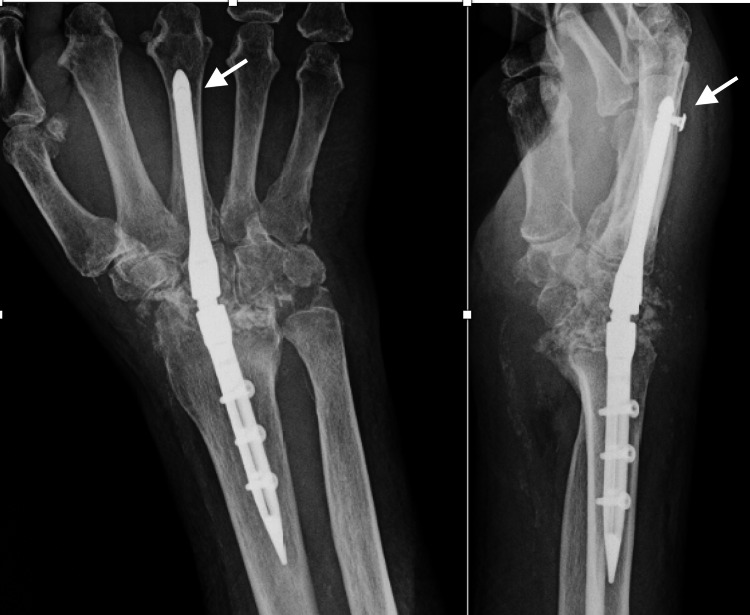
Immediate postoperative radiographs of Case 2 Posteroanterior and lateral views after total wrist arthrodesis using IMPLATE intramedullary nail and proximal row carpectomy. The white arrows show the proper positioning of the distal screw.
